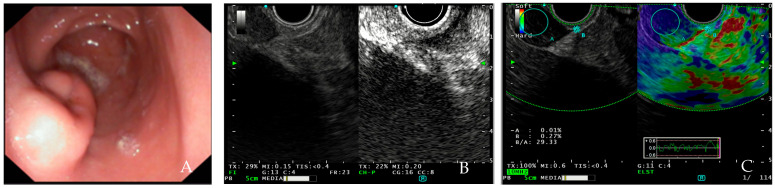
(A) EUS showing GIST in the antrum of stomach; (B) GIST in contrast-enhanced endoscopic ultrasound showing hypervascularization and displaying inhomogeneous contrast-uptake; (C) elastography of GIST located in the duodenum showing blue pattern. [source Division of Gastroenterology and Digestive Endoscopy, Humanitas Research Hospital—IRCCS, Rozzano, Milan].
